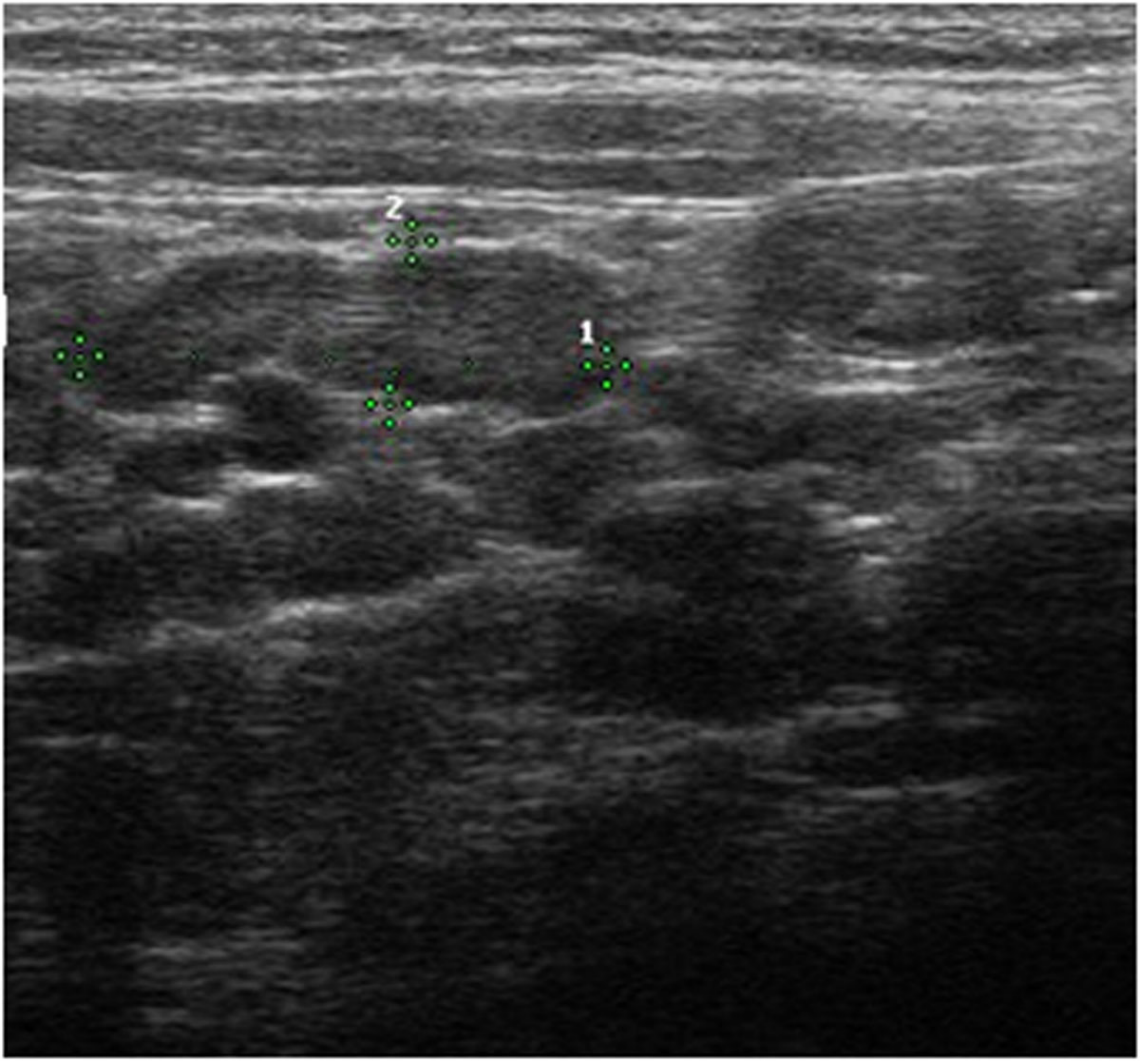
Ultrasound features of appendicitis. Other findings of appendicitis include enlarged mesenteric lymph nodes.
